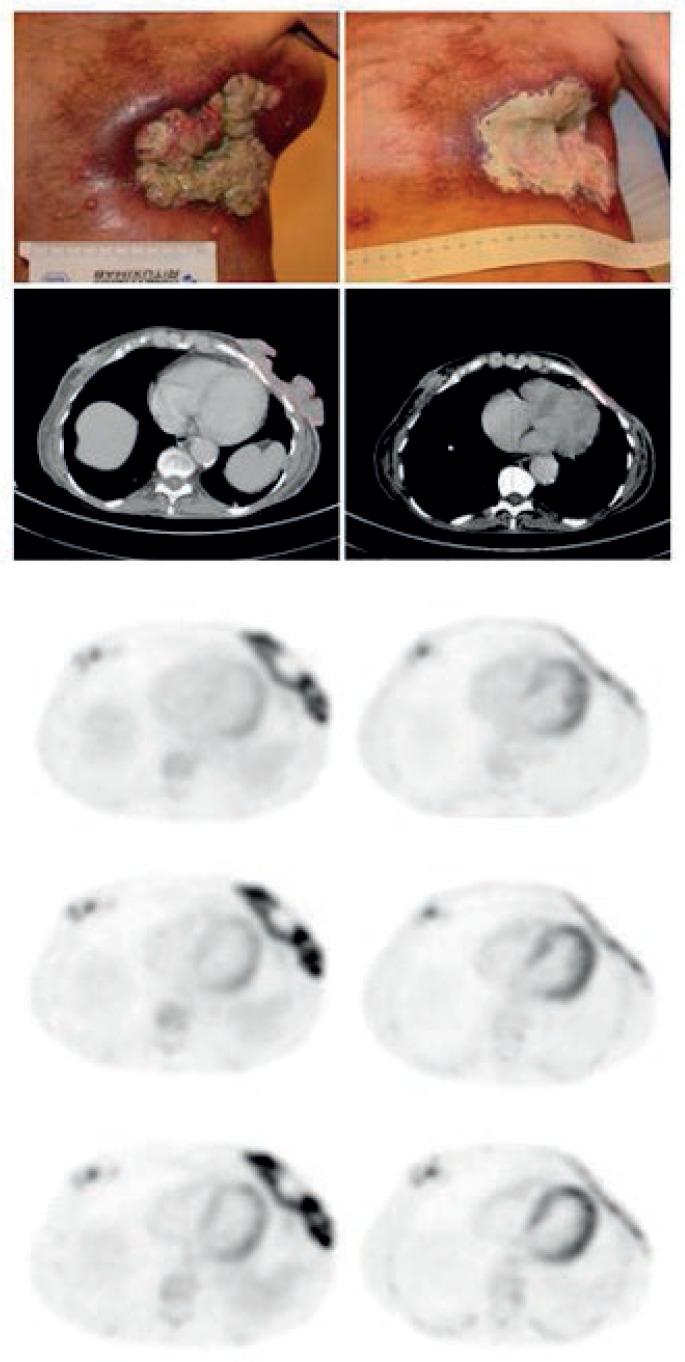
Large recurrence with varying depth and inflammation on the left chest wall of 68 y female. Left column shows from the top clinical presentation, CT-scan, PET scan at 60 min p.i., PET scan at 120 min p.i. and PET scan 180 min p.i. Right column. Same patients after one treatment. Change in SUVmax in target lesion at baseline compared to follow up was 29.7% at 60 min p.i., 71.2% at 120 min p.i., and 83.1% at 180 min p.i.
